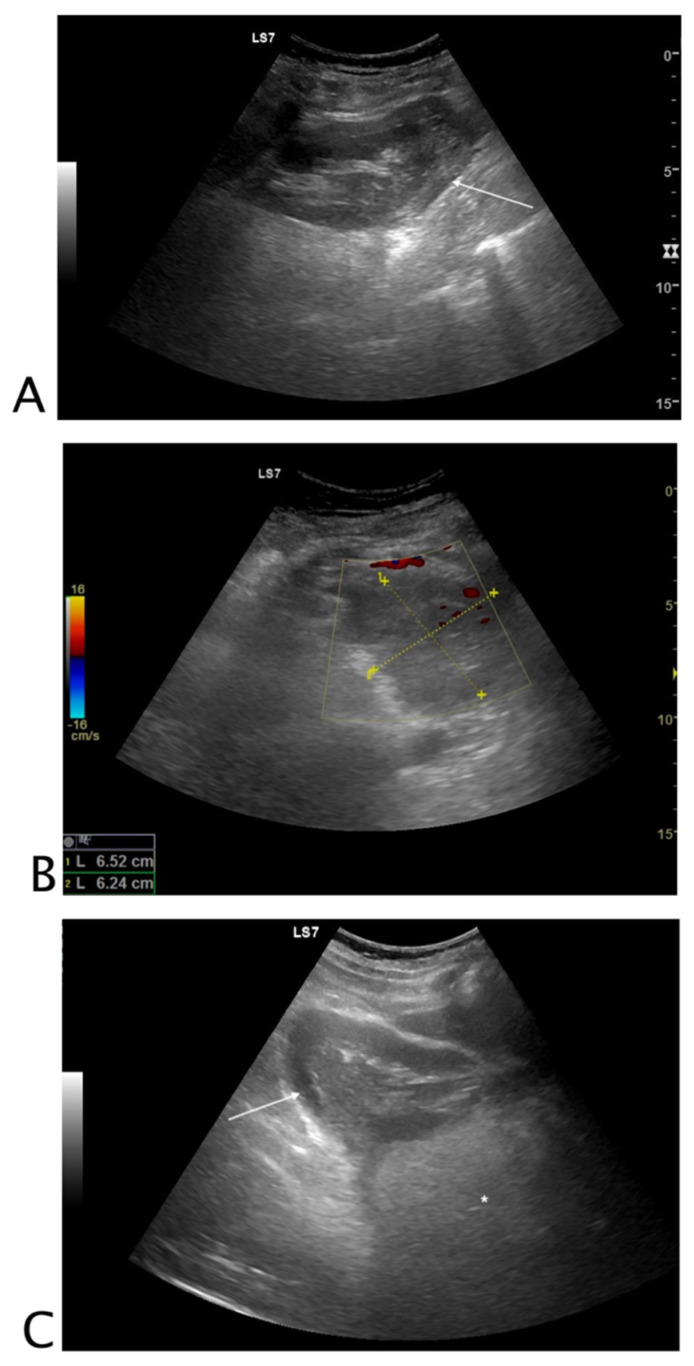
Black where it should not be. A 26-year-old female patient with abdominal tenderness and fainting. At ultrasound, an inhomogeneous subcapsular fluid collection (white arrow) was detected all around the right kidney (A). On the lower pole of the kidney, fluid collection was detected (B). On the upper pole of the right kidney, a hyperechoic mass (*) was seen, referable to angiomyolipoma (C). A suspected ultrasound diagnosis of hemo-retroperitoneum for spontaneous angiomyolipoma bleeding was formulated. The patient underwent CT angiography that confirmed active bleeding of the right kidney’s upper pole angiomyolipoma. The patient underwent endovascular arterial embolization. Final diagnosis: spontaneous bleeding of renal angiomyolipoma.
